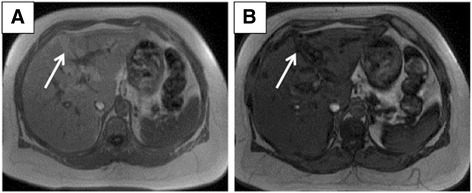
Axial images through the liver confirm subcapsular steatosis as seen on computed tomography in Fig. 1. a Axial T1-weighted in-phase gradient echo magnetic resonance imaging (repetition time/echo time = 150/4.4) shows areas of hyperintensity in the subcapsular region (*arrow*). b Axial T1-weighted opposed-phase gradient echo magnetic resonance imaging (repetition time/echo time = 150/2.2) shows signal loss in the corresponding subcapsular regions (*arrow*)
